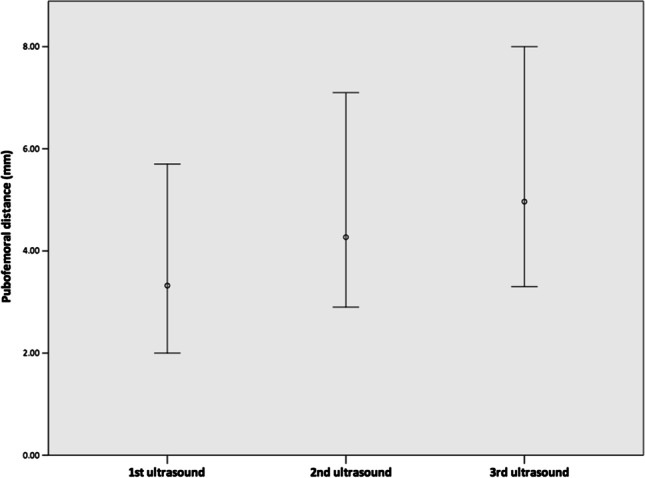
Distribution of the pubofemoral distance measured at three serial hip ultrasounds
